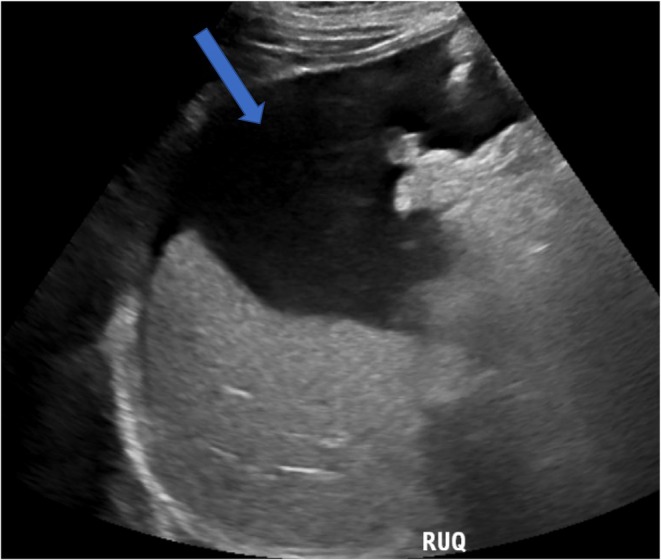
Abdomen ultrasound moderate‐to‐large ascites (blue arrow).
